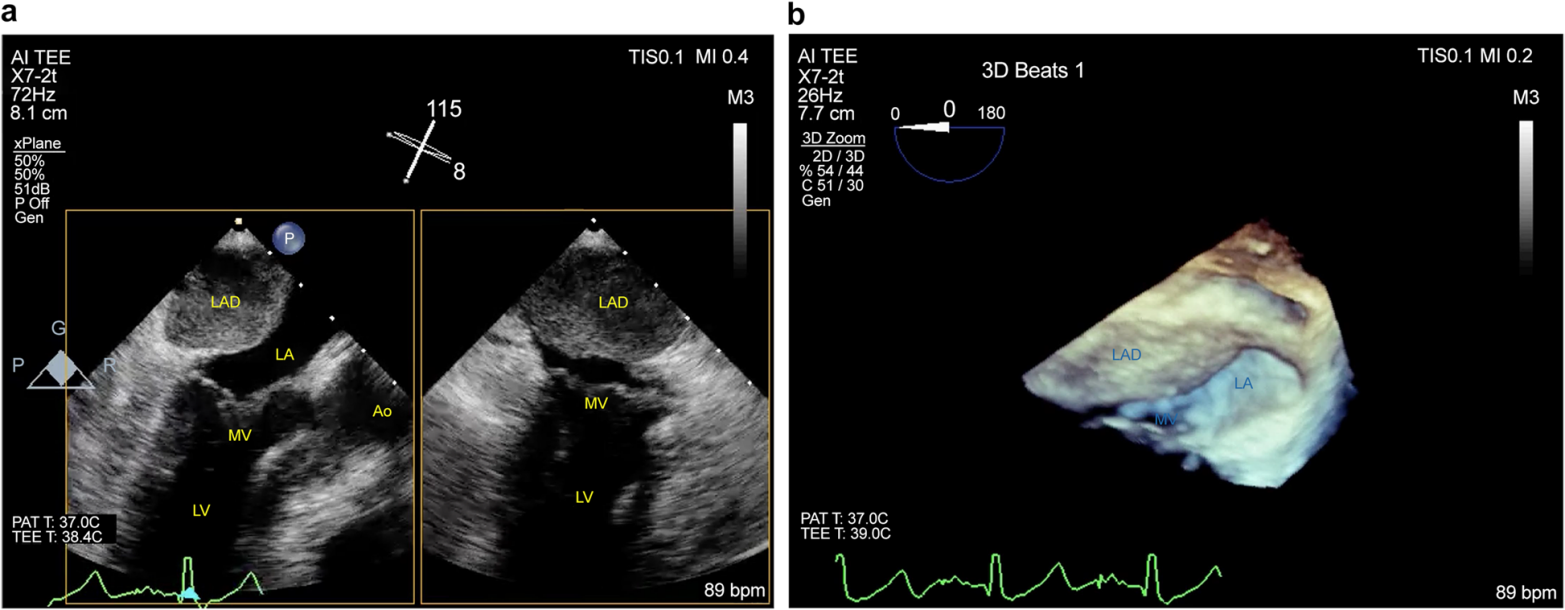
Transesophageal echocardiography (TEE) during total arch replacement (TAR). a 2D-TEE exhibited the left atrial dissection (LAD) located at the posterior wall of the left atrium (LA). b The LAD and mitral valve (MV) were partly visible on 3D TEE. The LAD contains a thrombus. *Ao* aorta, *LV* left ventricle
